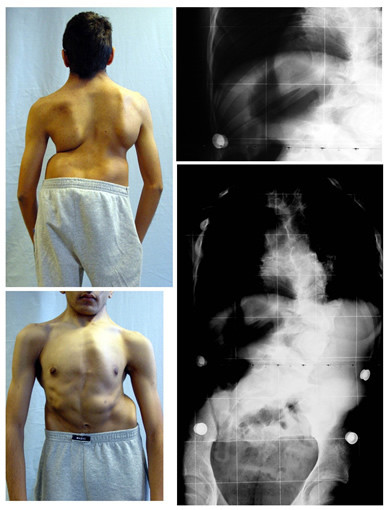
Patient with failure of segmentation with clinical and radiological improvement due to conservative treatment. This 15 years old boy presented at the age of 9, with congenital scoliosis with rib synostosis due to failure of segmentation, before entering the pubertal growth spurt. During his last follow - up, his clinical appearance demonstrates a severe deformity. The radiographs demonstrate a scoliotic curve of 59 degrees with Risser sign 4. VC was 1.640 ml, 33% of the predicted value.
